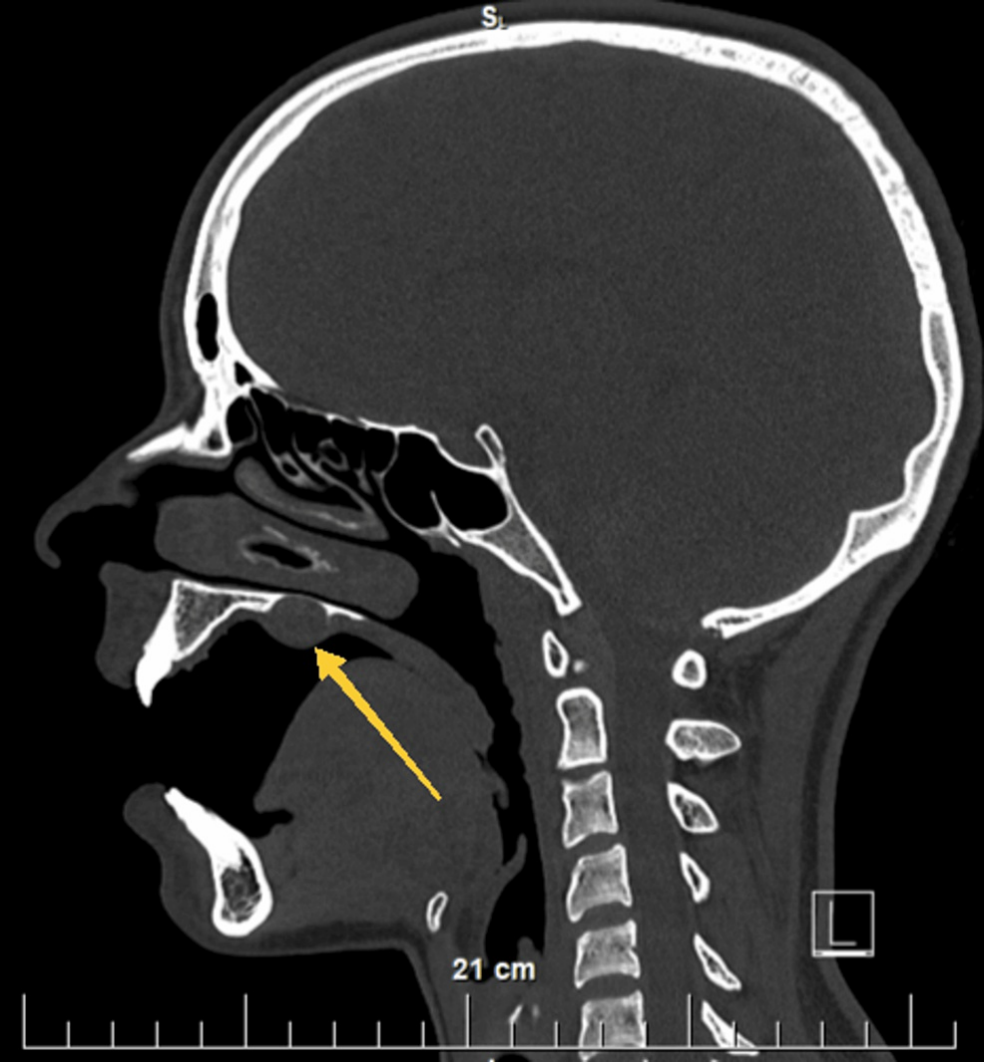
Sagittal CT section of the head after intravenous administration of contrast material. A yellow arrow shows the location of the lesion on the right hard palate and the bony plate thinning
